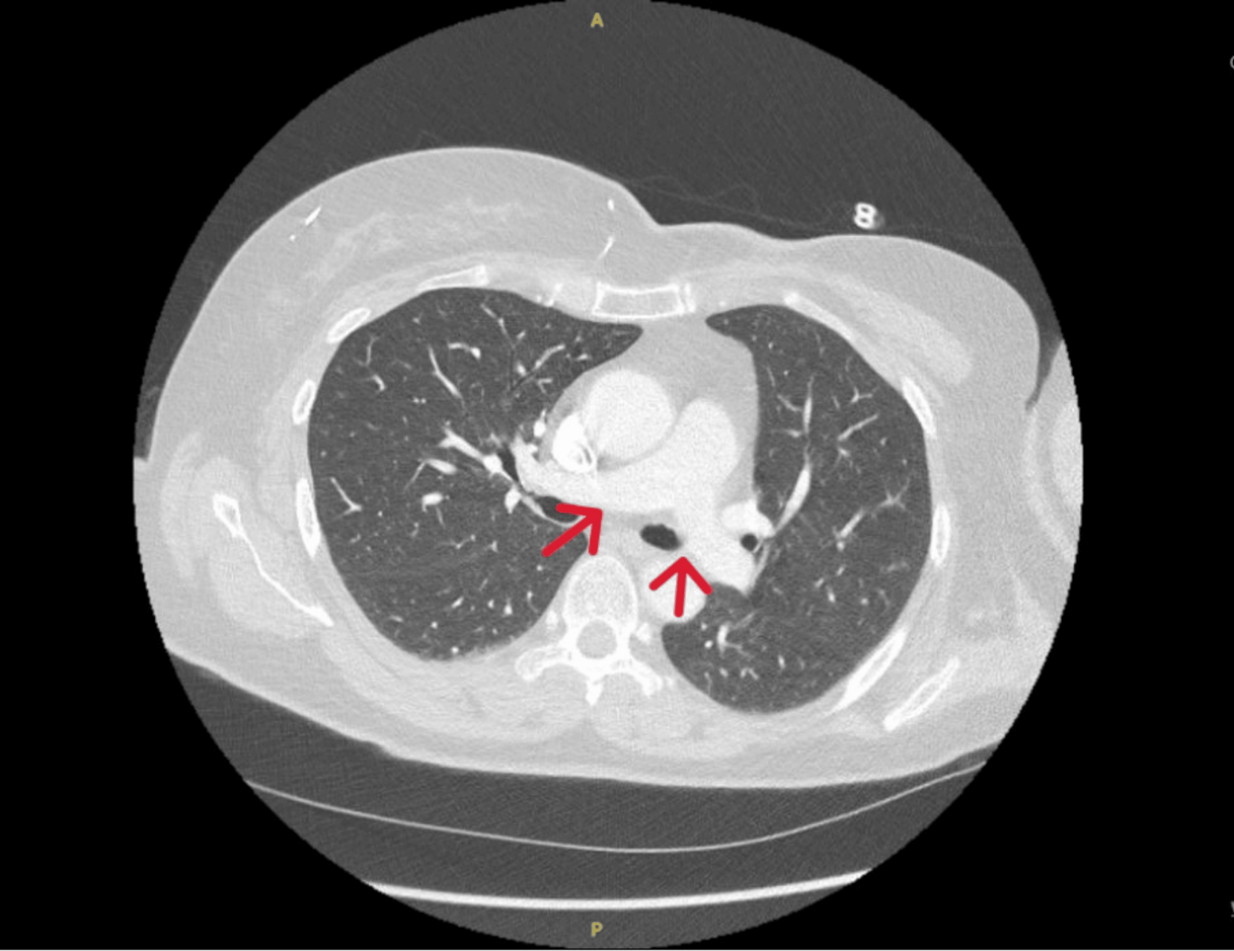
CTPA excluding the possibility of PE CTPA: computed tomography pulmonary angiogram, PE: pulmonary embolism
